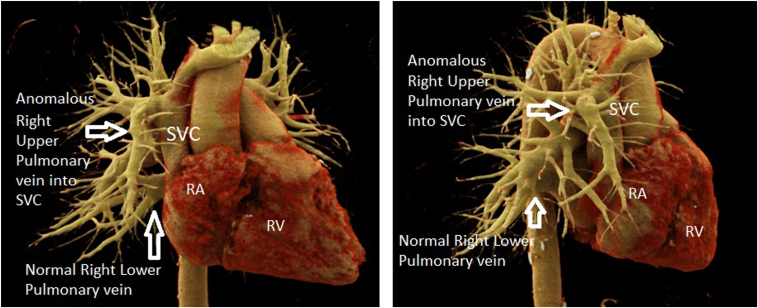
Three-dimensional computed tomography reconstruction showing an anomalous right upper pulmonary vein draining into the superior vena cava (SVC). RA = right atrium; RV = right ventricle.
